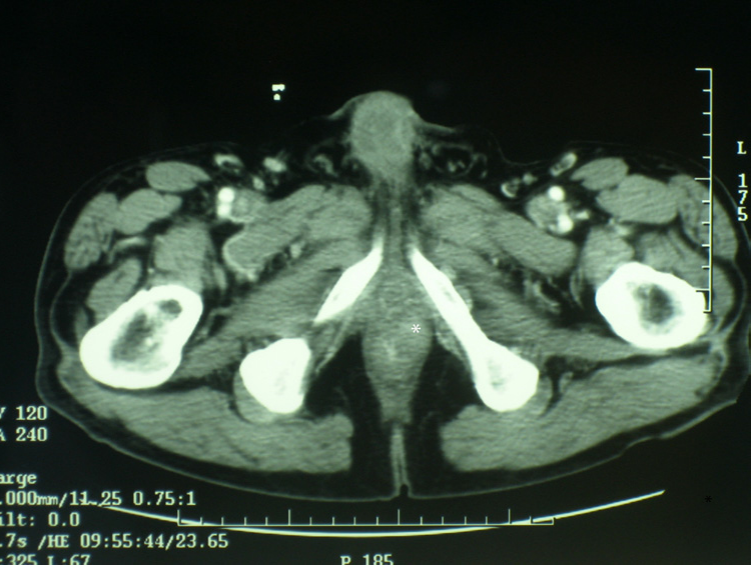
CT scan shows the mass (*) along the left anterior aspect of the anal canal.
